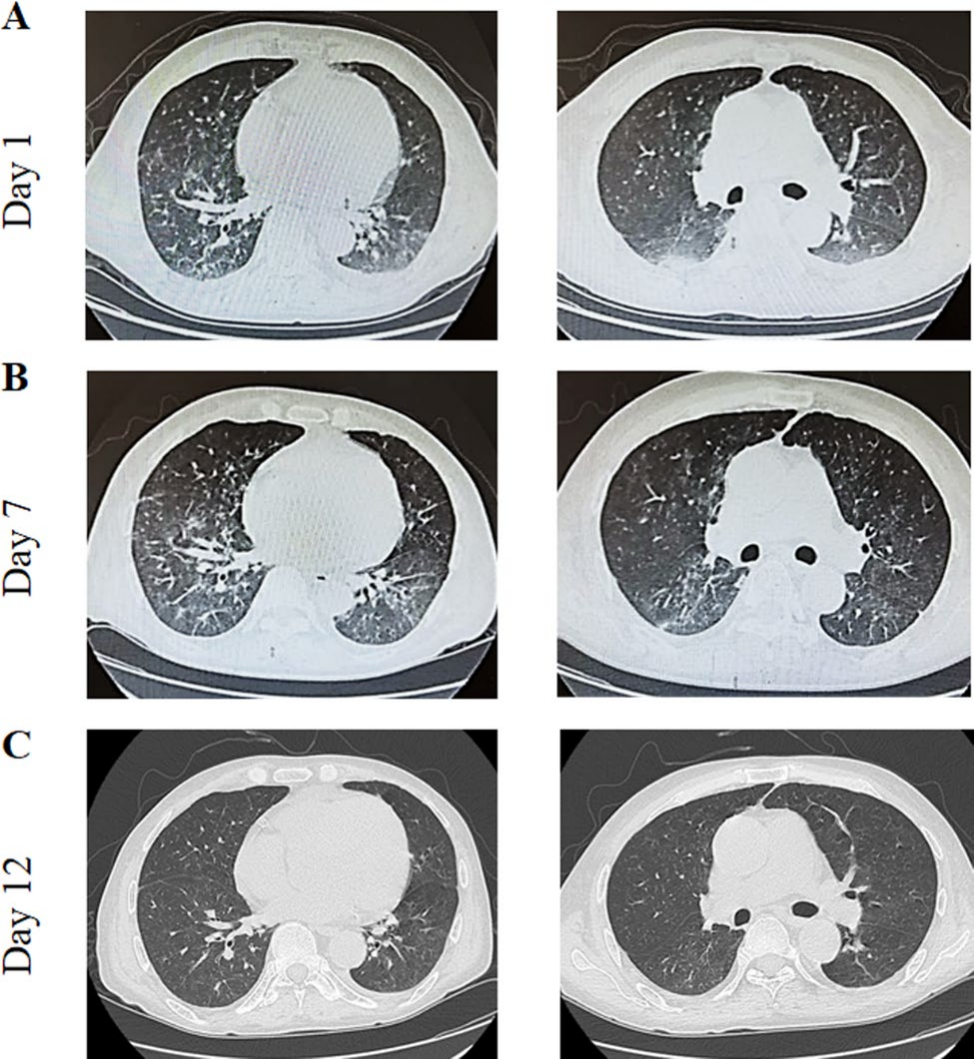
Case 2-Computed tomography images at different hospital days
